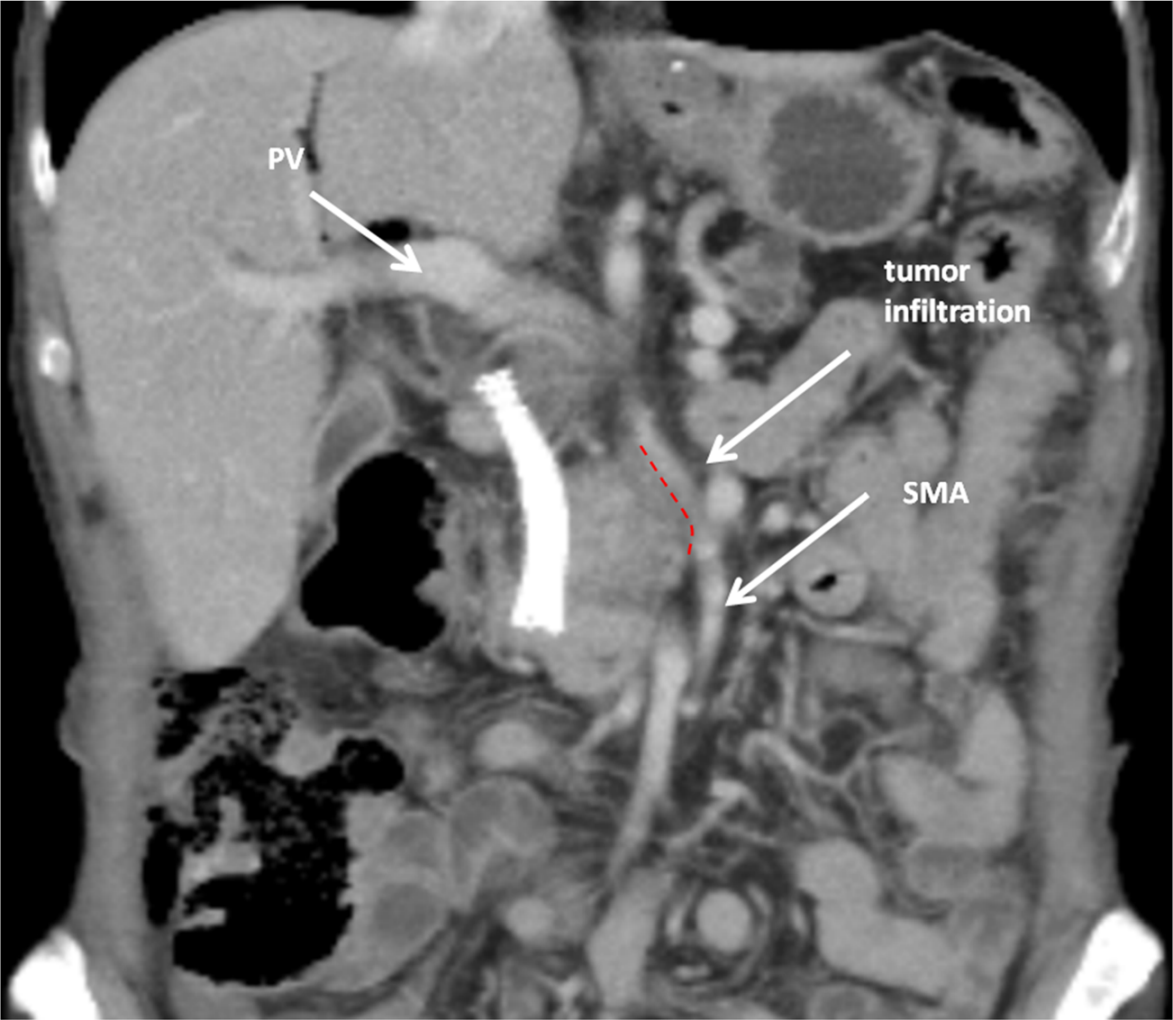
The post neo-adjuvant CT scan shows unchanged tumor encasement of the superior mesenteric. *SMA* = superior mesenteric artery. *PV* = portal vein
